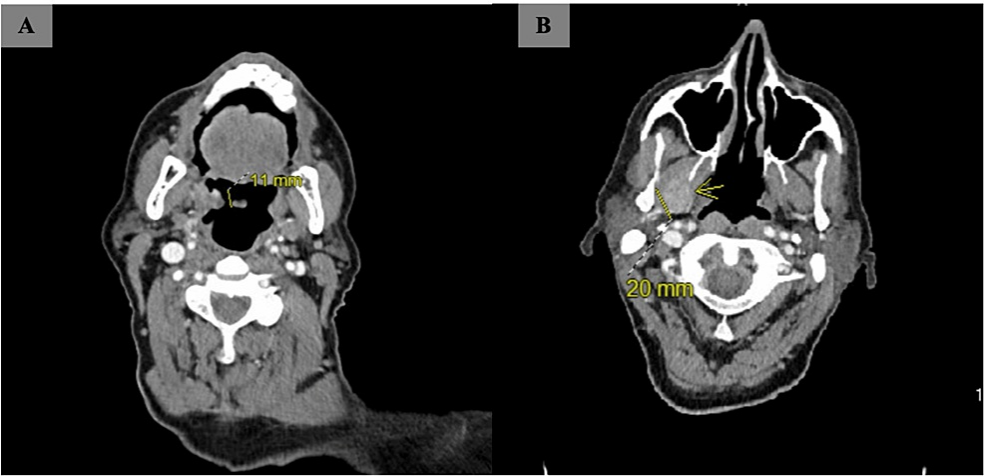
Pre-treatment computed tomography of the head and neck with contrast Pre-treatment computed tomography of the head and neck with contrast; (A) Exophytic nodule 1.1 cm involved the right palatine tonsil, (B) Enhancing lesion 2 cm in the right masticator space affected the medial and lateral pterygoid muscles
